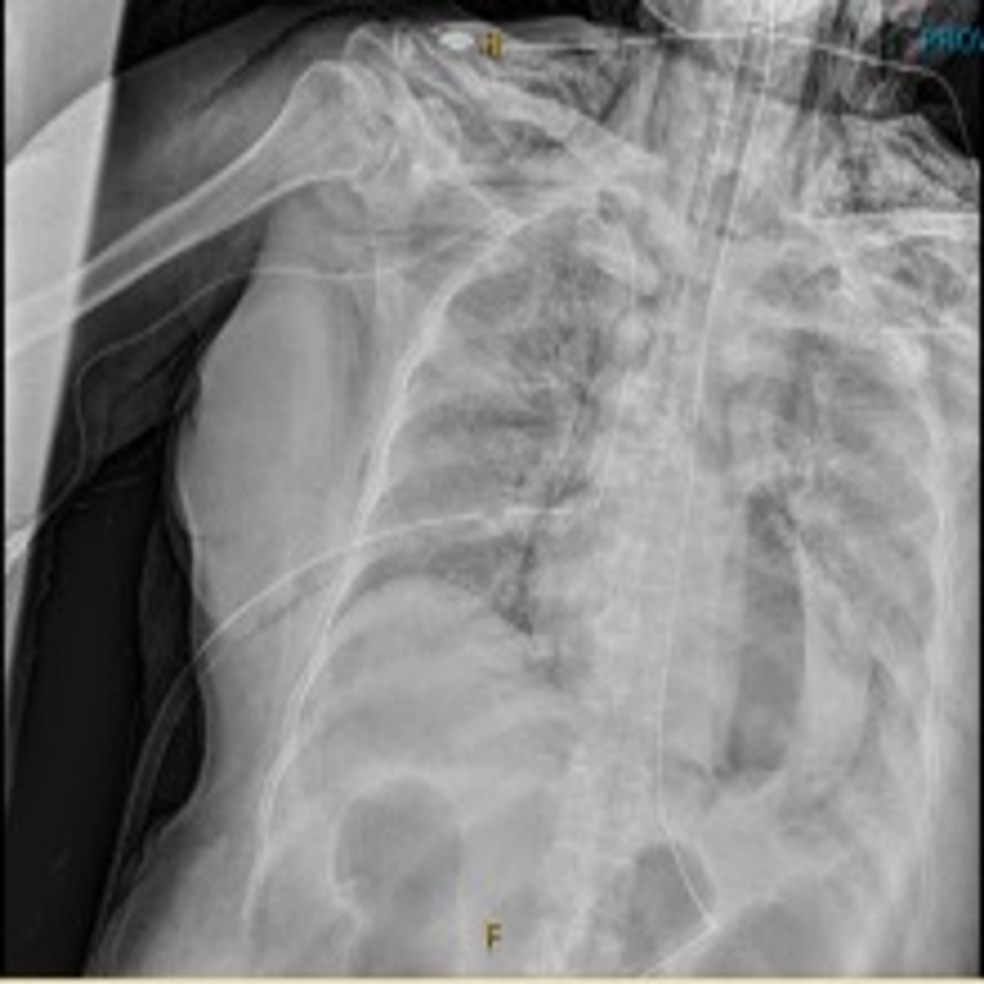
Chest X-ray after first chest tube. Chest X-ray showing persistent right pneumothorax despite initial chest tube placement requiring second chest tube.
